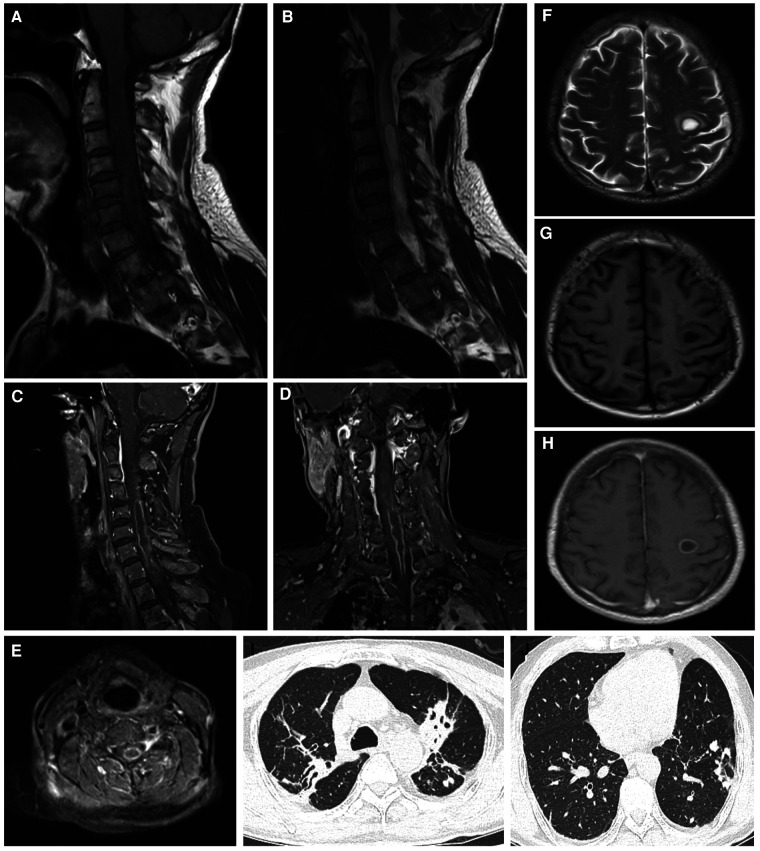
The spinal cord was imaged using MRI, including sagittal views in T1WI (A), T2WI (B), and contrast-enhanced T1WI (C) additionally, coronal views in contrast-enhanced T1WI were obtained (D), along with axial views in contrast-enhanced T1WI (E) furthermore, the brain was examined using MRI, specifically axial views in T1WI (F), T2WI (G), and contrast-enhanced T1WI images were acquired as well (H). Chest CT scans revealed suspicious tuberculosis foci (I, J). MRI, magnetic resonance imaging; CT, computerized tomography; T1WI, T1-weighted imaging; T2WI, T2-weighted imaging.
